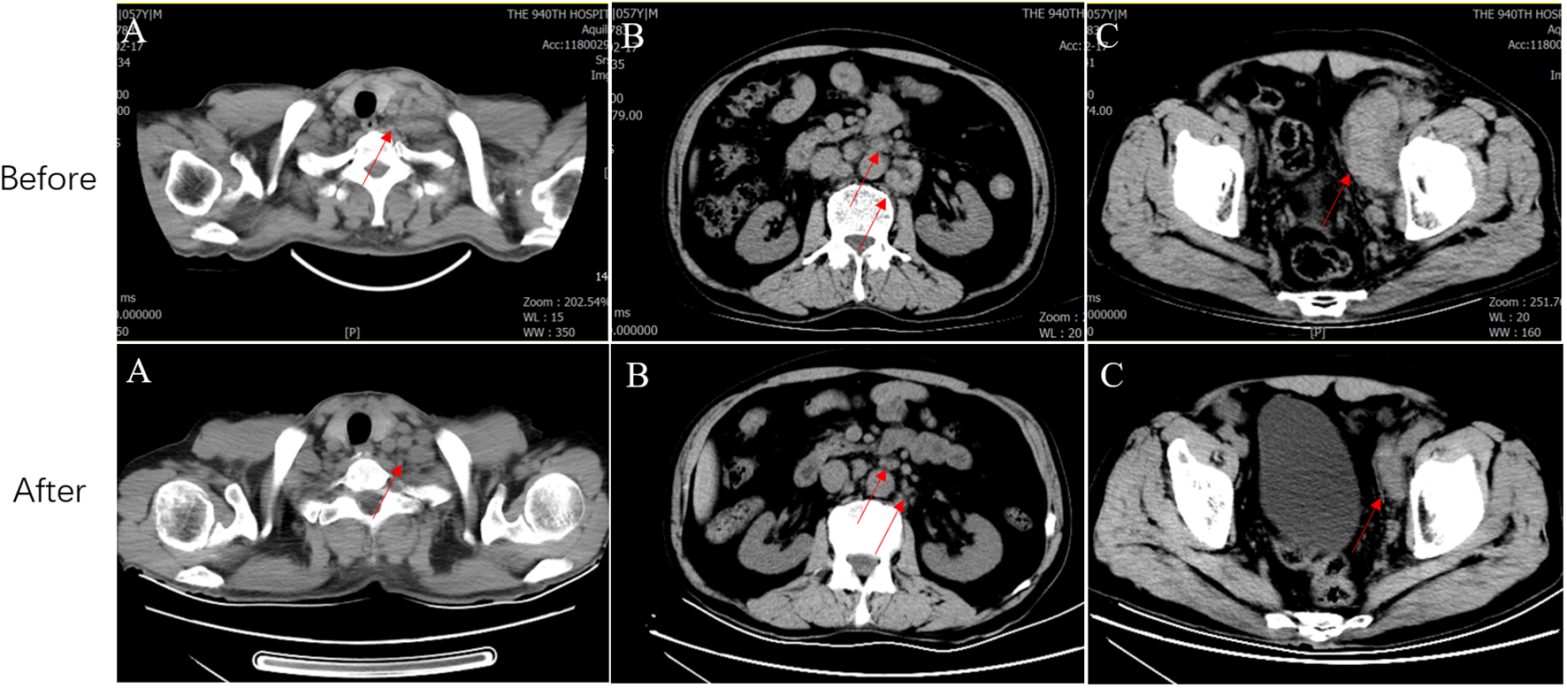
CT before and after systemic therapy. The computed tomography shows multiple enlarged lymph nodes in the neck (A), retroperitoneal (B) and pelvic (C) regions (Red arrows represent metastatic lymph nodes). CT, Computed tomography.
